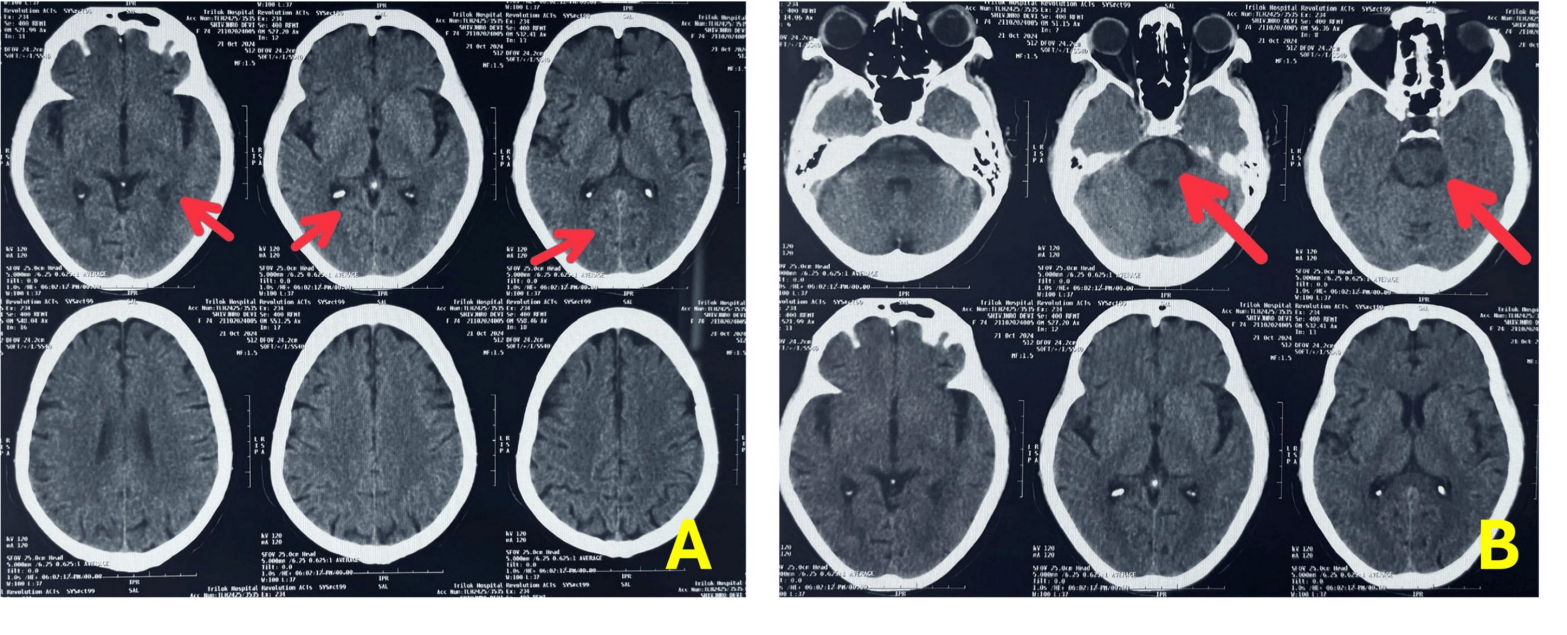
(A) Axial non-contrast computed tomography (NCCT) of the brain at the level of the lateral ventricles showing bilateral periventricular white matter hypodensities (red arrows), consistent with chronic small-vessel ischemic changes. (B) Axial NCCT at the pontine level demonstrating a hypodense lesion (HU 7-11) in the left pons (red arrows).
